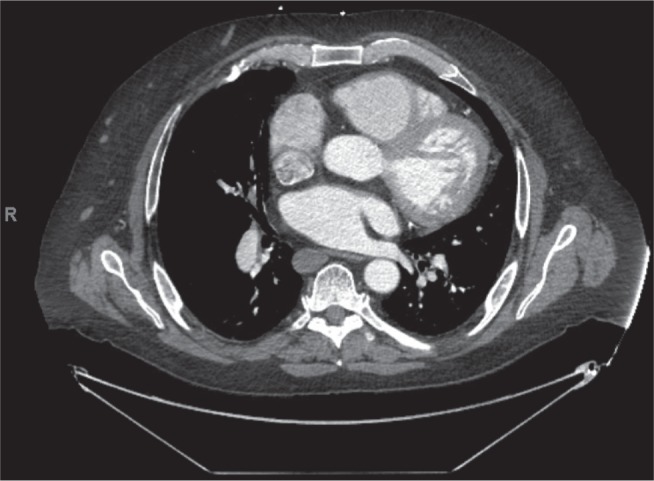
Chest CT with contrast demonstrating the SVC feeding into the right atrium. The descending aorta is seen, but the IVC is not visualized.
